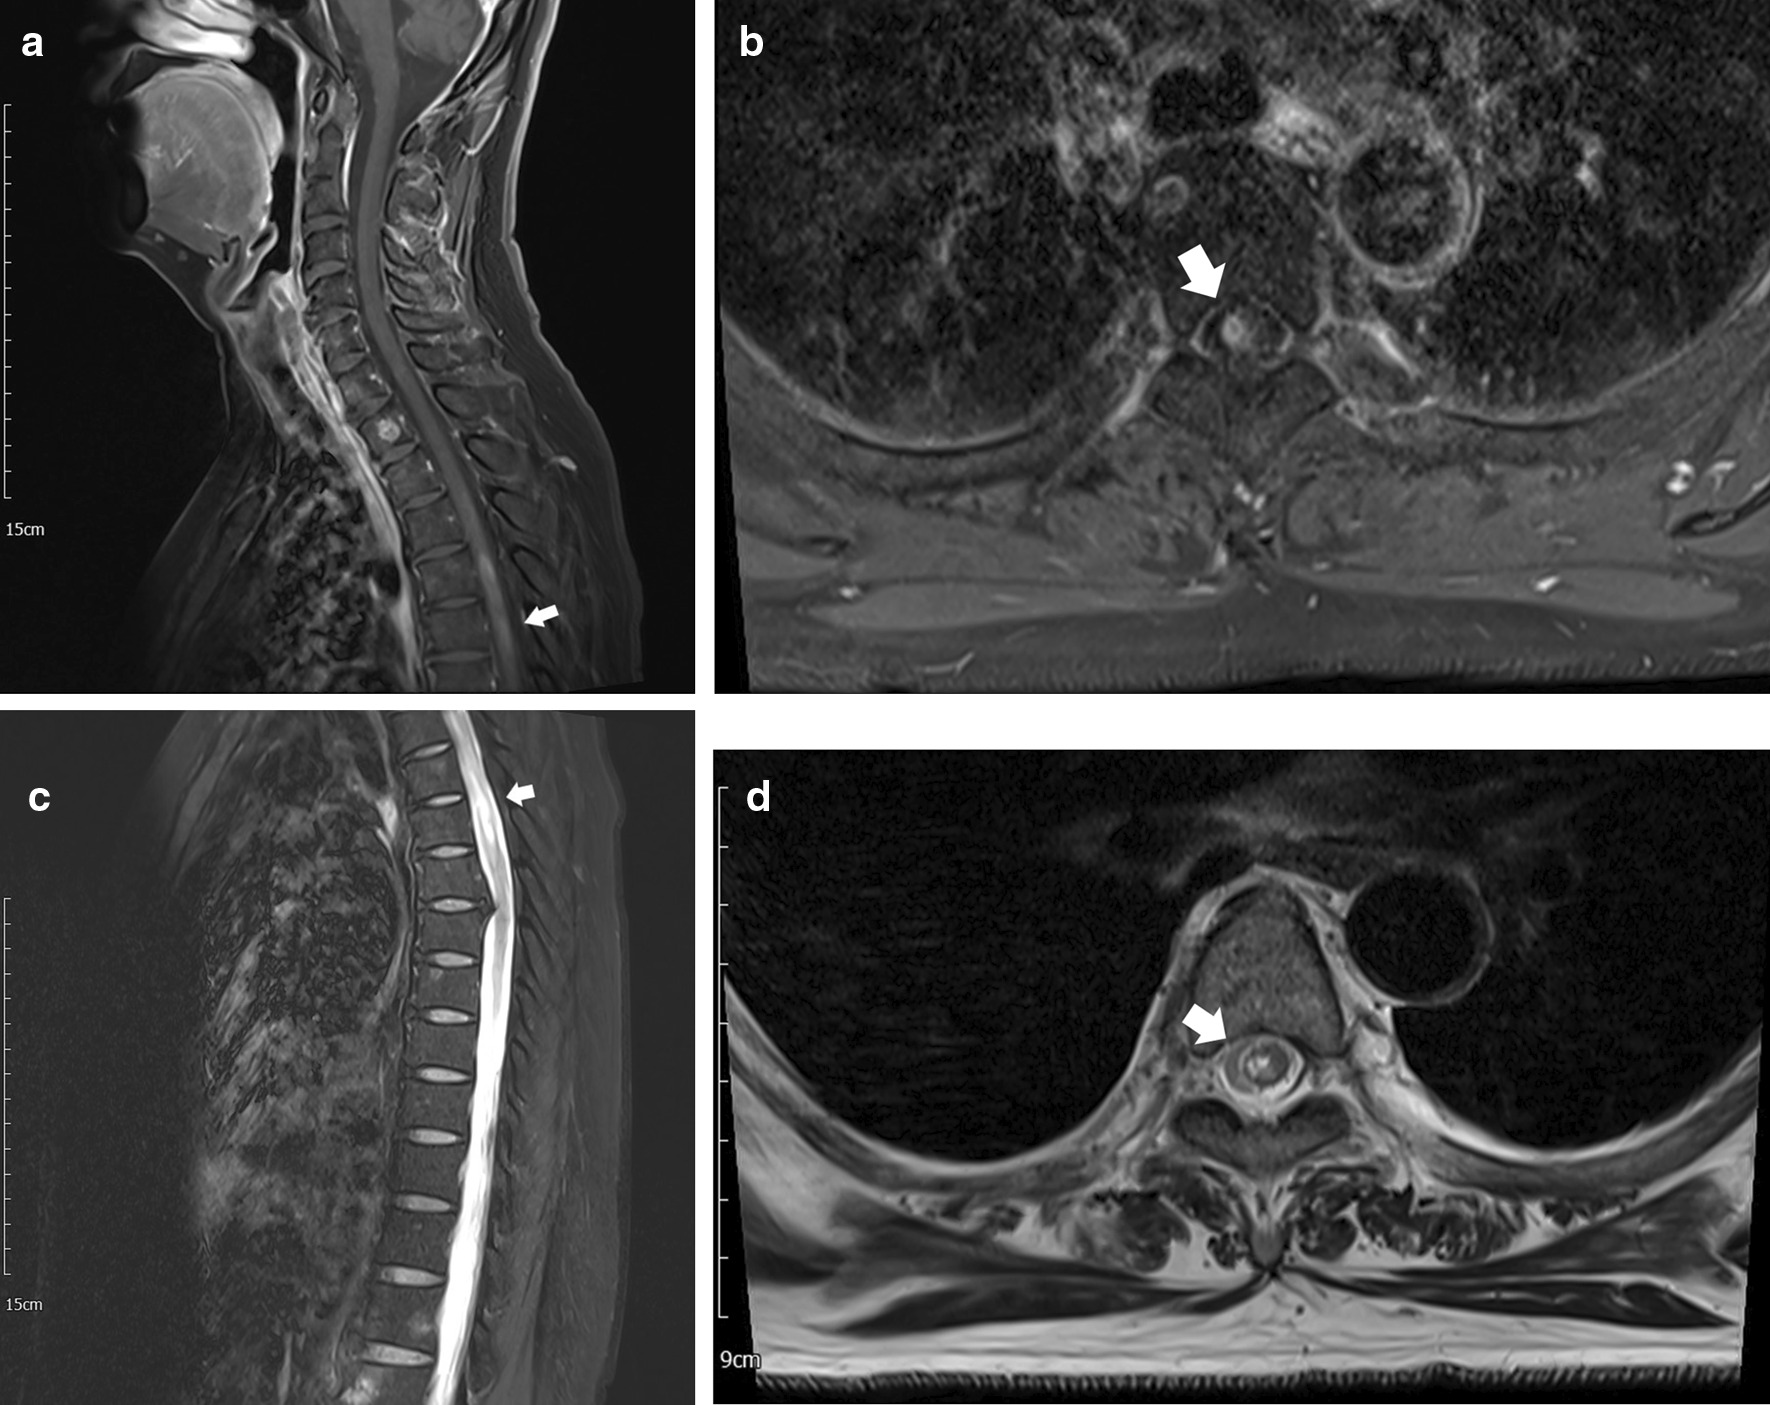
Preoperative magnetic resonance images of the patient
